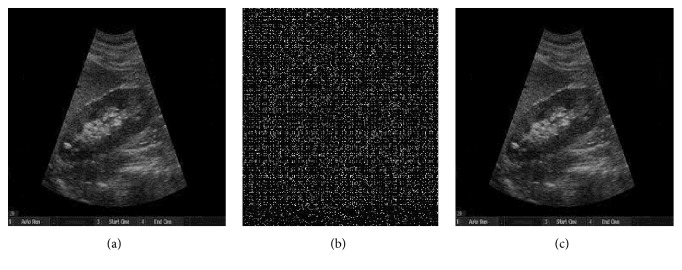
(a) Original MRI image; (b) recovered image with slightly modified initial conditions. (c) Recovered image with exact initial conditions.
